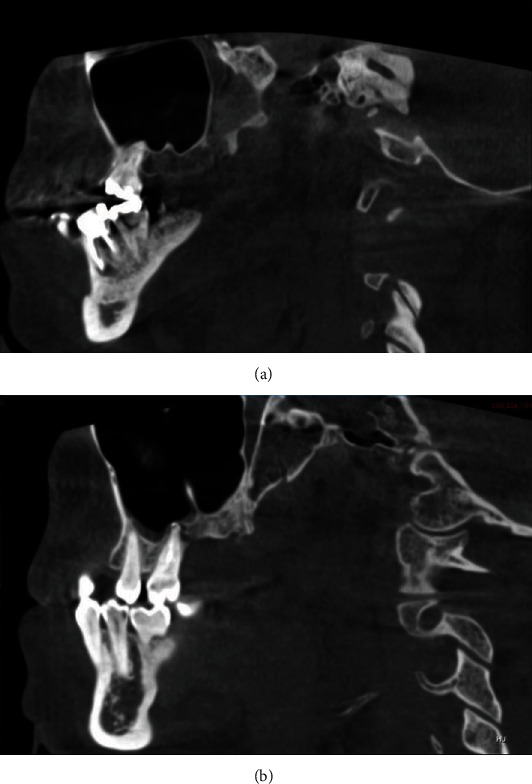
(a) Sagittal CBCT section showing presence of a small partial septation above surgical site. (b) Sagittal CBCT section showing large partial septation above surgical site.
